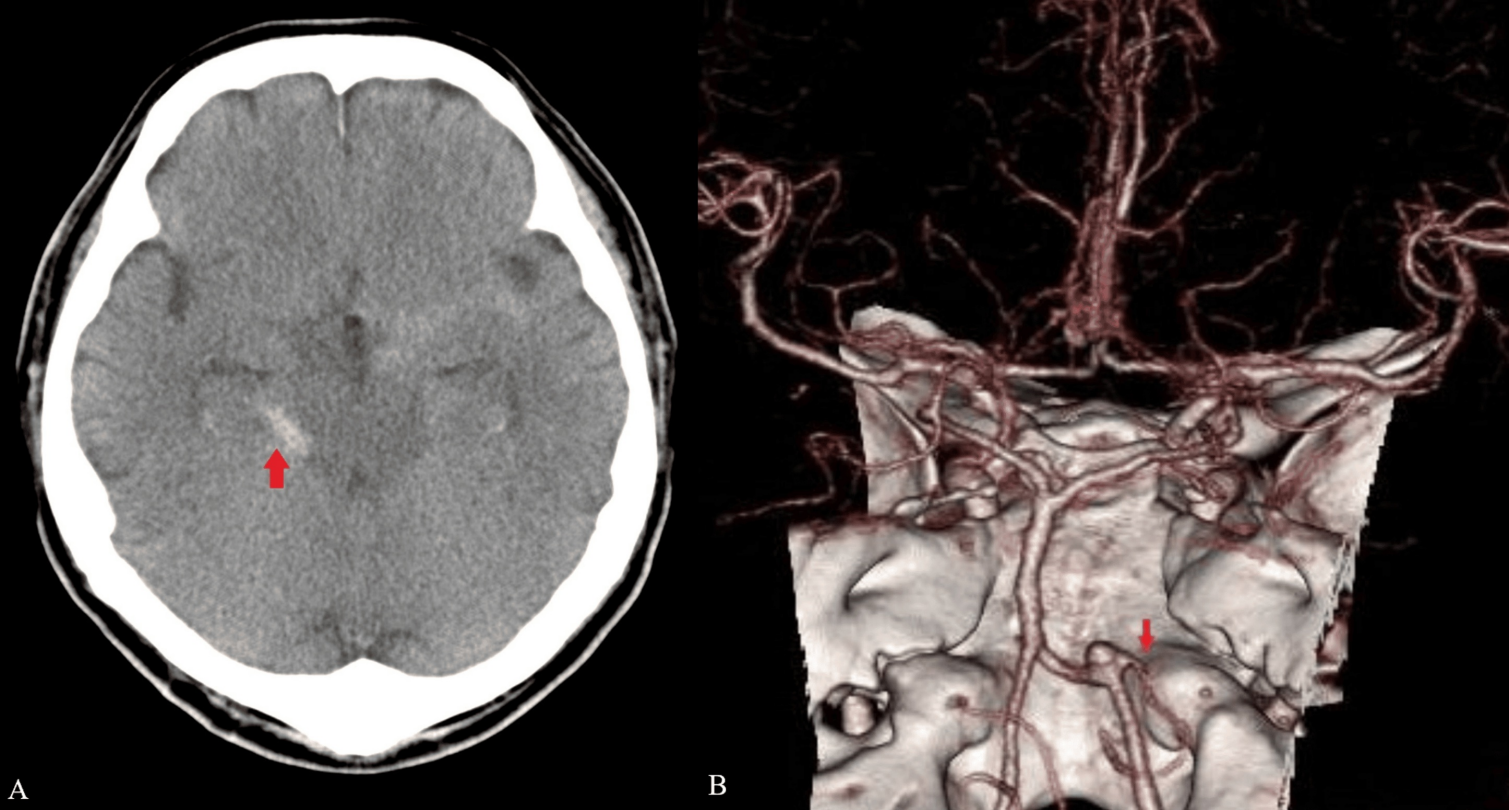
Preoperative head computed tomography and three-dimensional computed tomography angiography. (A) Computed tomography of the head showed Fisher 2 subarachnoid hemorrhage (arrow). (B) Three-dimensional computed tomography angiography demonstrating right vertebral artery-PICA aneurysm. Note the PICA originated from the neck of the aneurysm (arrow). PICA: posterior inferior cerebellar artery
